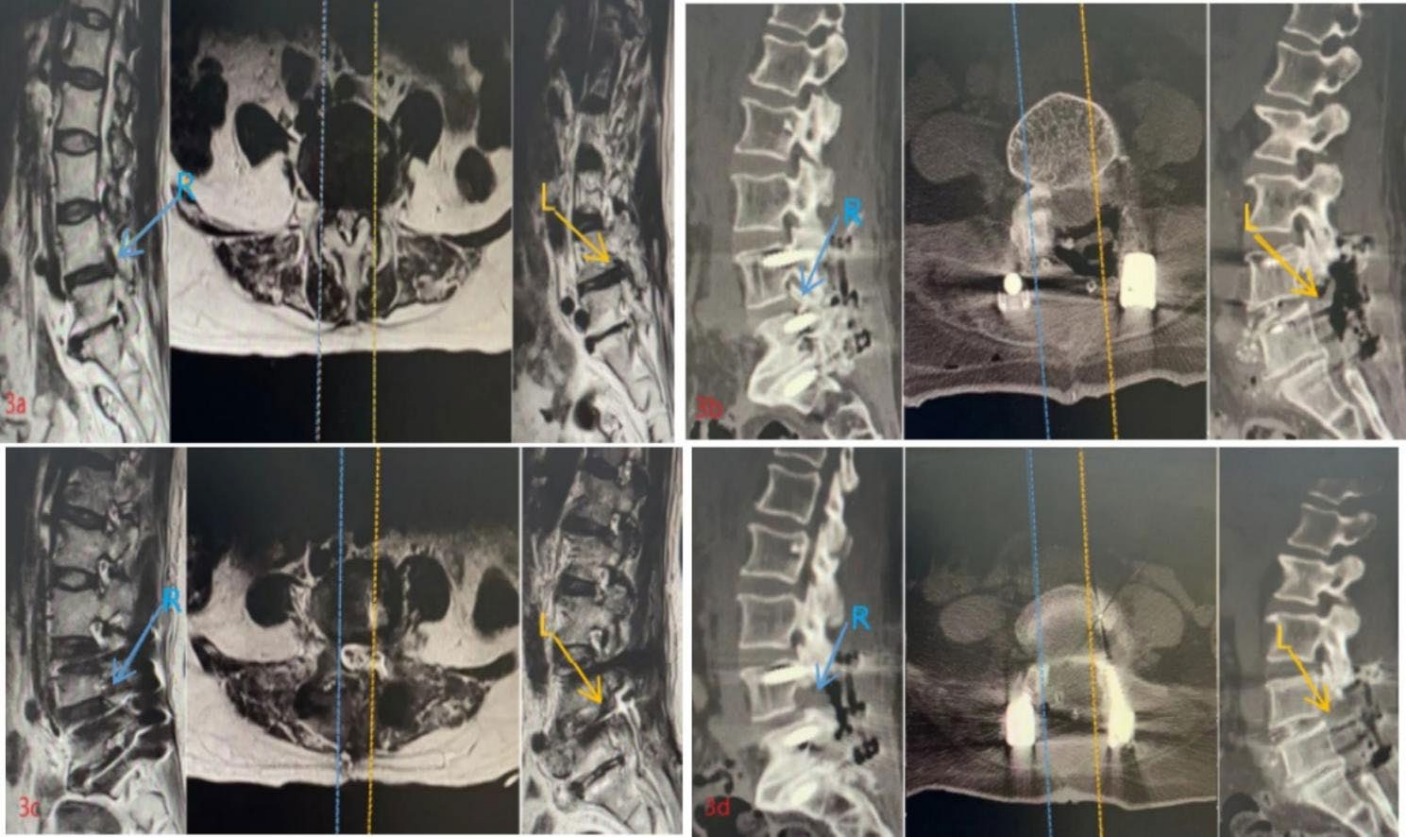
A 56-year-old female patient was admitted to the hospital due to low back pain with left lower limb pain and numbness for 5 years, which had been aggravated for 1 week. Lumbar decompression and interbody fusion were performed through the left intervertebral foramen of L4 and 5, and no preventive decompression was performed on the right side. On the fifth day after the operation, the patient had grade 2 right ankle dorsiflexion muscle strength, grade 1 right hallux dorsiflexion muscle strength, and shallow sensation of the right hallux toe skin. The symptoms were not significantly improved after dehydration and conservative treatment. Subsequently, lumbar CT and MRI examinations were performed. Considering the dislocation of the right superior articular process of the lower vertebral body, emergency nerve root release was performed, and postoperative recovery was acceptable. 3a, L4/5 bilateral intervertebral foramen severe stenosis; 3b,3c, Lumbar decompression and interbody fusion was performed through the left intervertebral foramen of L4/5, and no preventive decompression was performed on the right side during the operation; 3d, After the operation, the symptoms of right intervertebral foramen stenosis occurred, and nerve root release was performed. The right inferior articular process of L4 and the right superior articular process of L5 were removed with bite forceps
